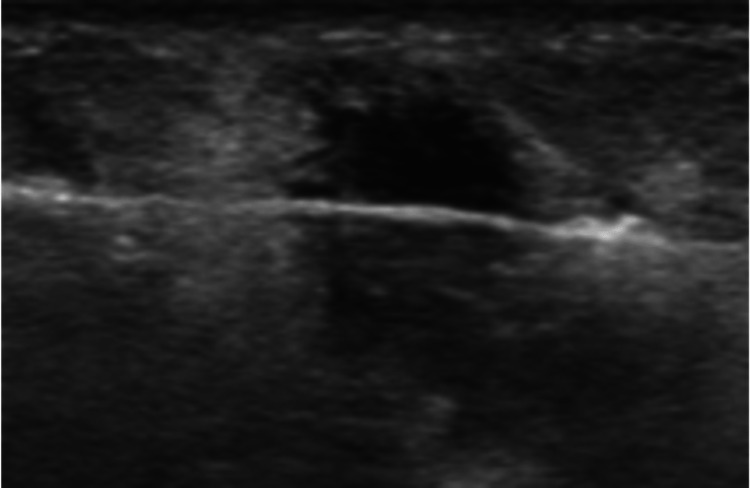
Ultrasound-guided biopsy was performed
